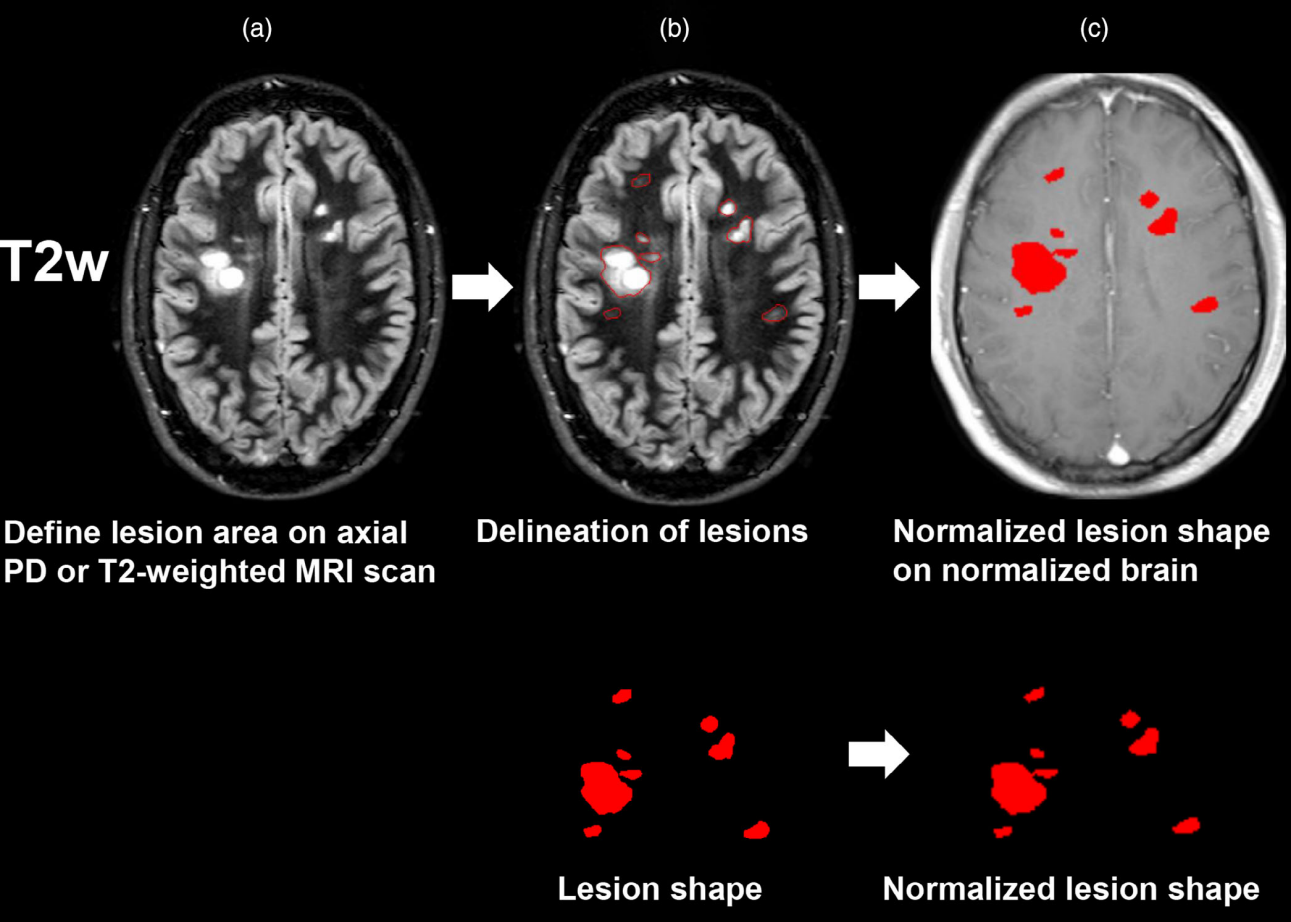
Process of lesion delineation and spatial normalization. (a) T2‐weighted MR images in original space. (b) The T2‐weighted axial image was used to define the area of T2‐hyperintense MS‐lesions shown as a red contour and below the red lesion shape. (c) The lesion shape, the T2‐weighted as well as the T1‐weighted MRI scans were then transformed into stereotaxic space which generated the normalized map. The normalized lesion map was also applied to the normalized T1‐weighted brain to qualitatively demonstrate the accuracy of image normalization [Color figure can be viewed at http://wileyonlinelibrary.com]
